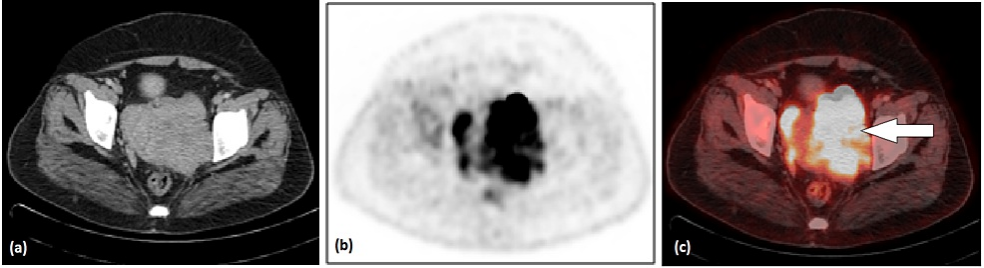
Pelvic CT (a), PET (b) and PET CT fusion (c) axial images showing hypermetabolic cervical/upper vaginal mass (white arrow) with parametrial extension and left pelvic sidewall involvement.
